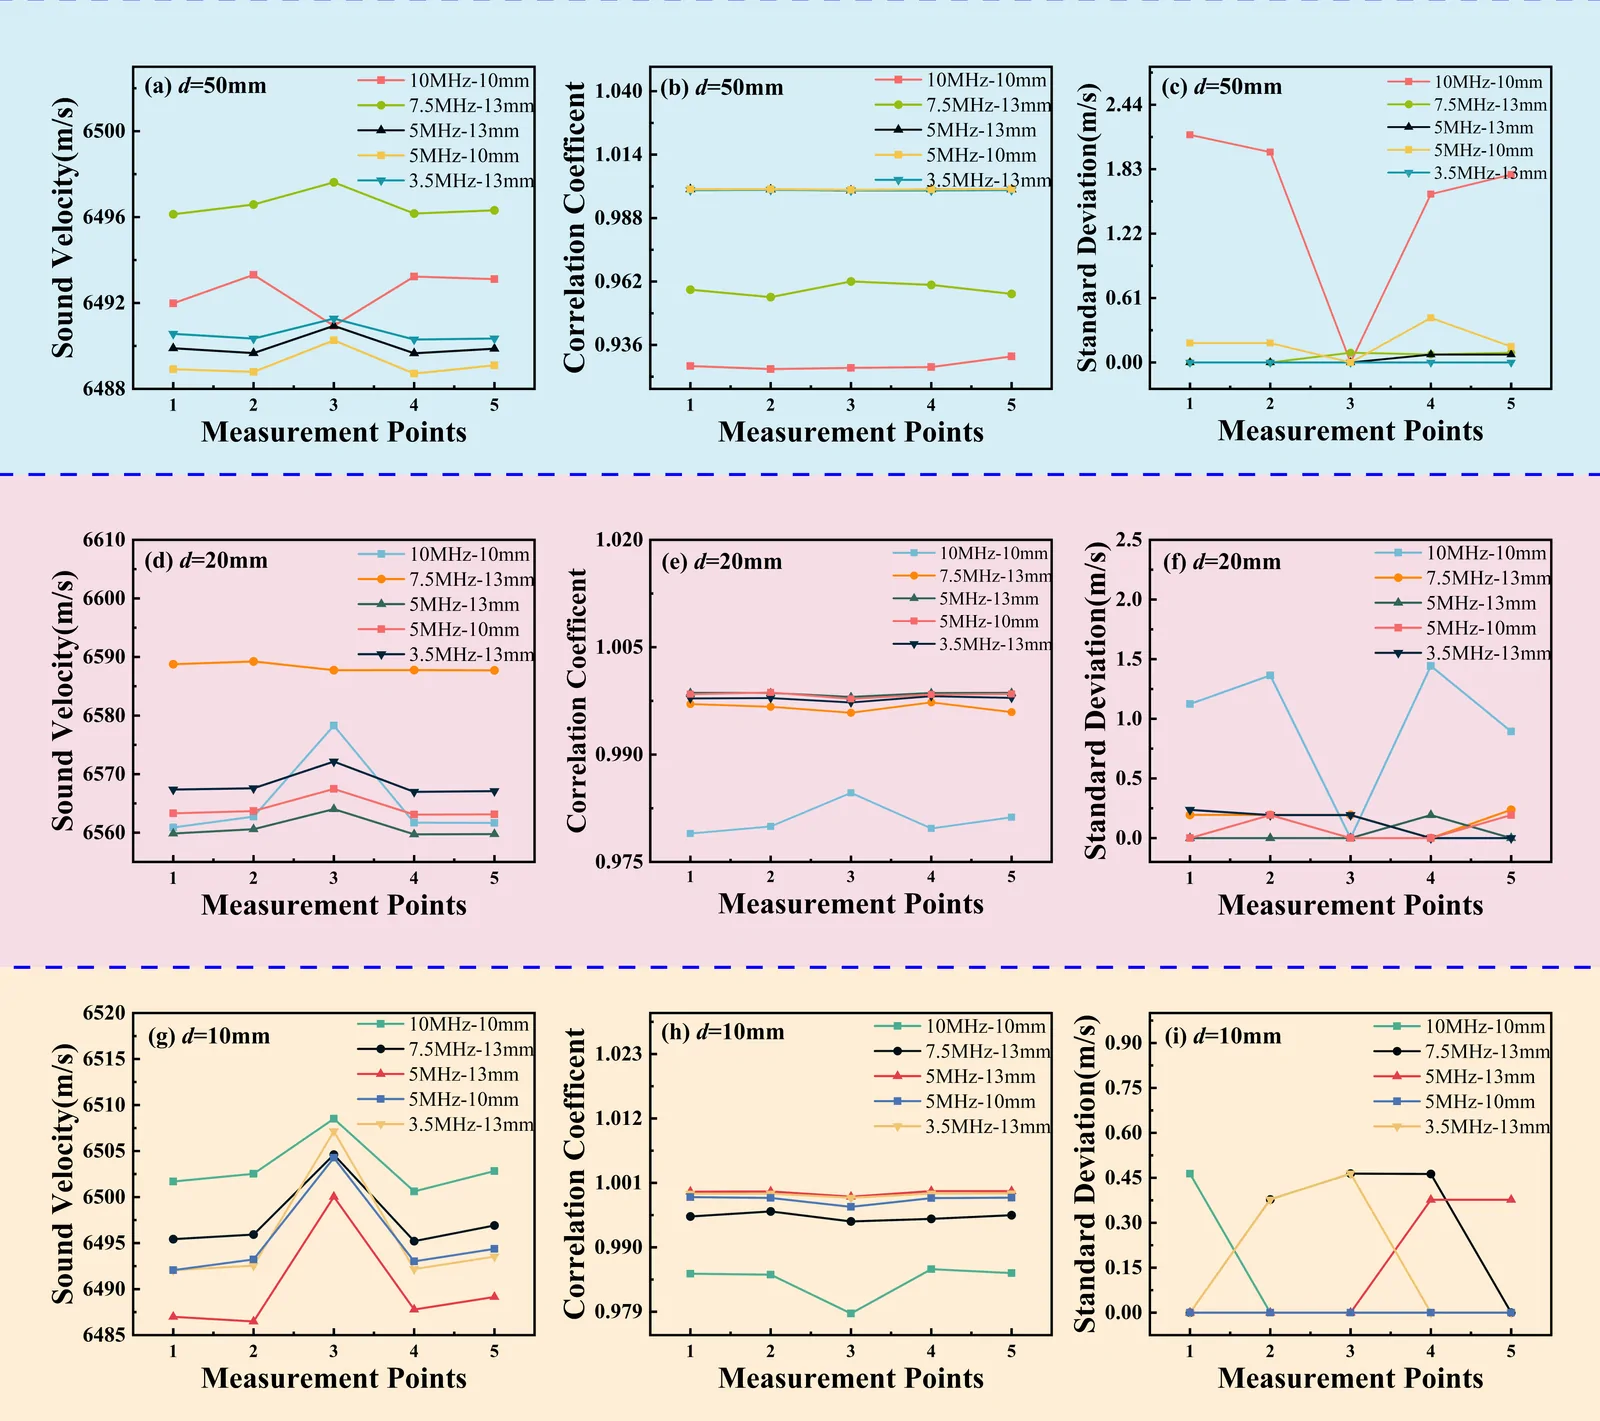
Comprehensive comparative chart of measurement results obtained with different ultrasonic transducers: (a–c) measurement results for samples with thicknesses of 50 mm; (d–f) measurement results for samples with thicknesses of 20 mm; (g–i) measurement results for samples with thicknesses of 10 mm.
